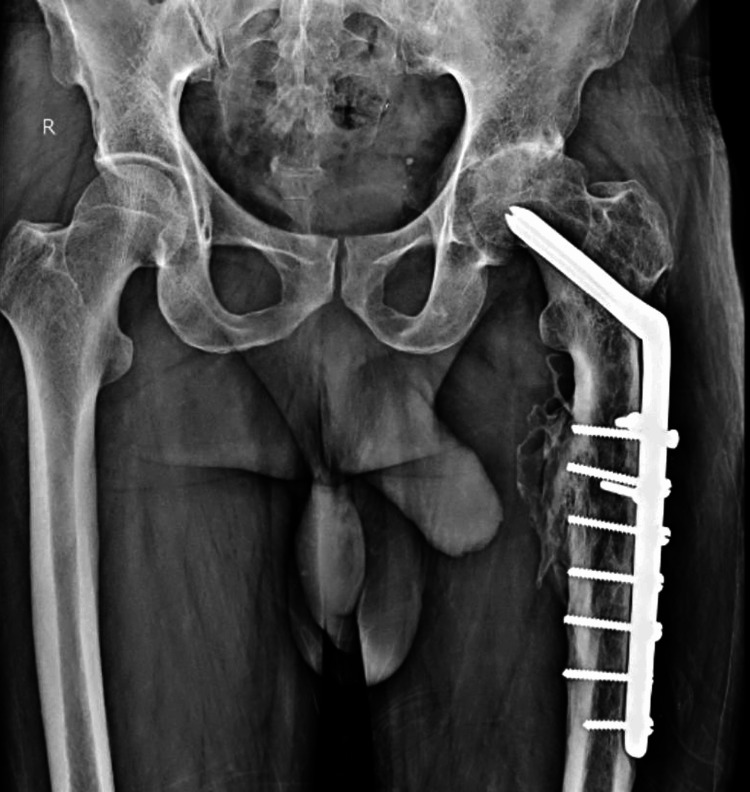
X-ray of the united subtrochanteric femur fracture on the left side with the implant in situ.
